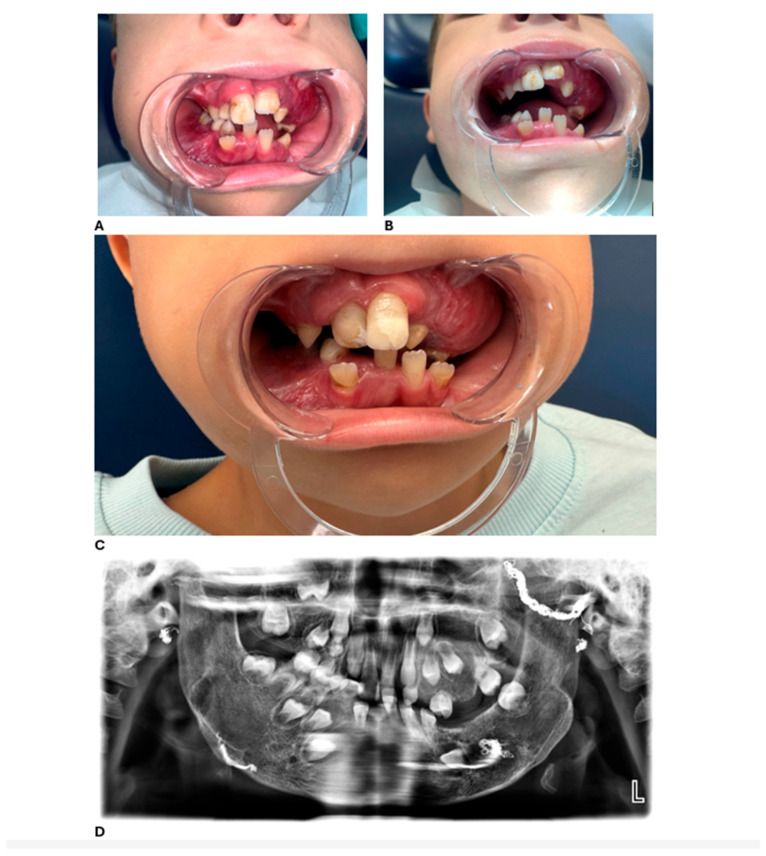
Intraoral findings at 6 and 7 years of age. (A) Dysplasia of upper central incisors, ectopic teeth, and gingival overgrowth at 6 years of age. (B) Alveolar bone overgrowth in the upper left molar region at 6 years of age. (C) Alveolar bone overgrowth, ectopic teeth, and malocclusion at 7 years of age. (D) Panoramic radiograph at 7 years of age.
